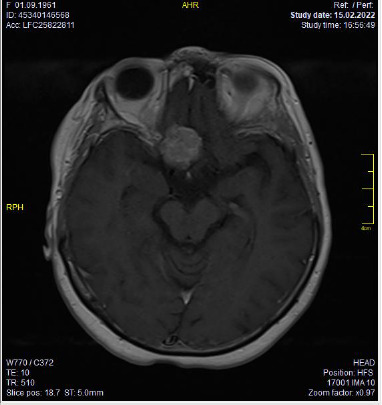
A contrast-enhanced magnetic resonance imaging (MRI) of the brain and orbits of the patient
